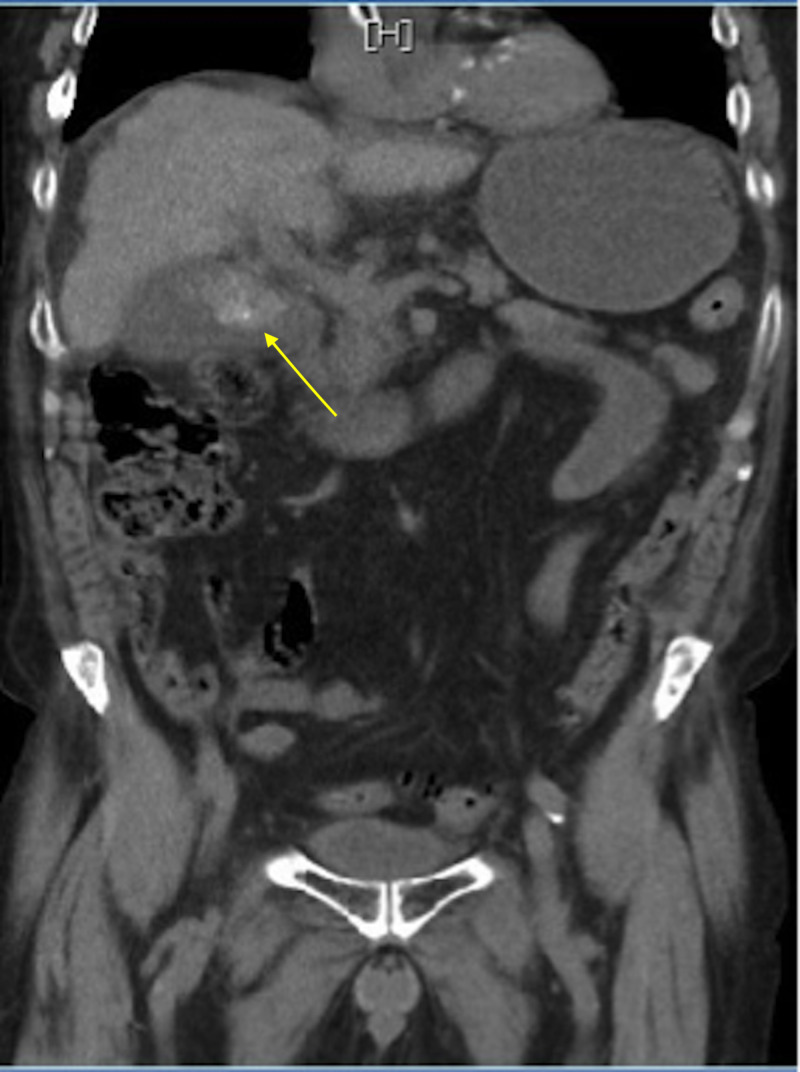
CT of the abdomen and pelvis without contrast Cholelithiasis with enlarged gallbladder or liver mass
